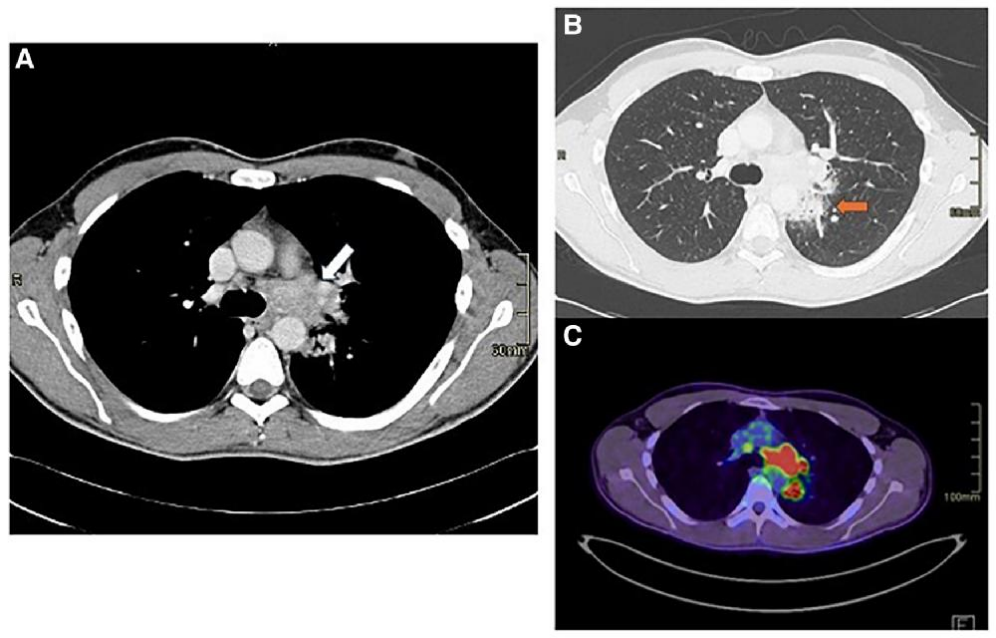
CT scan (*A* and *B*) confirmed the presence of a mass in the aortopulmonary window, extending towards the left pulmonary hilum and oesophagus (indicated by the arrow, *A*) and of a thickening area in the left pulmonary upper lobe (indicated by the arrow, *B*). PET-CT scan showing the tracer hyperaccumulation in the mass and the pulmonary lesion (*D*).
